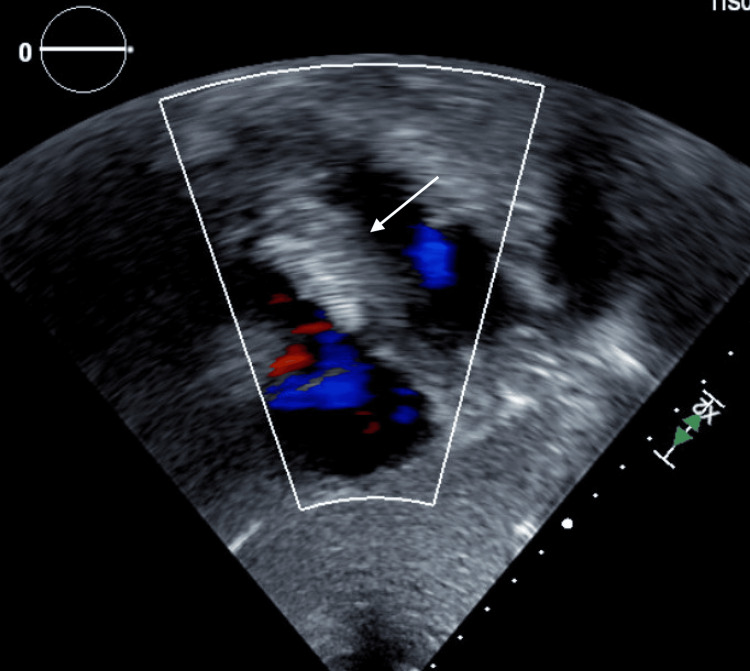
Transthoracic echocardiogram demonstrating the 26mm Amplatzer Septal Occluder positioned across the atrial septum with no residual shunting.
